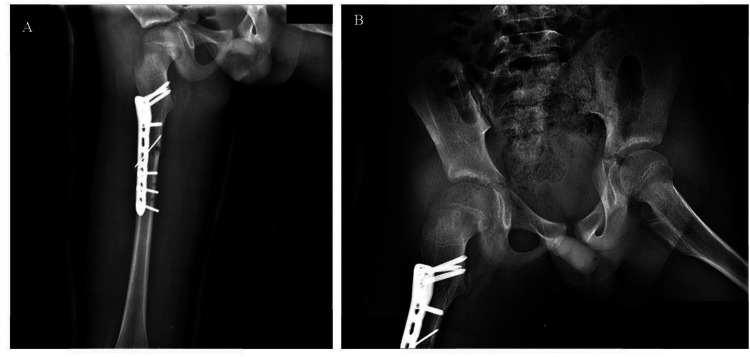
A x-ray demonstrated the healing of the right femoral shaft fracture at 1-month follow-up, with the right femoral head located in the acetabulum; B x-ray showed the right femoral head remains within the acetabulum on the 2-month follow-up.
